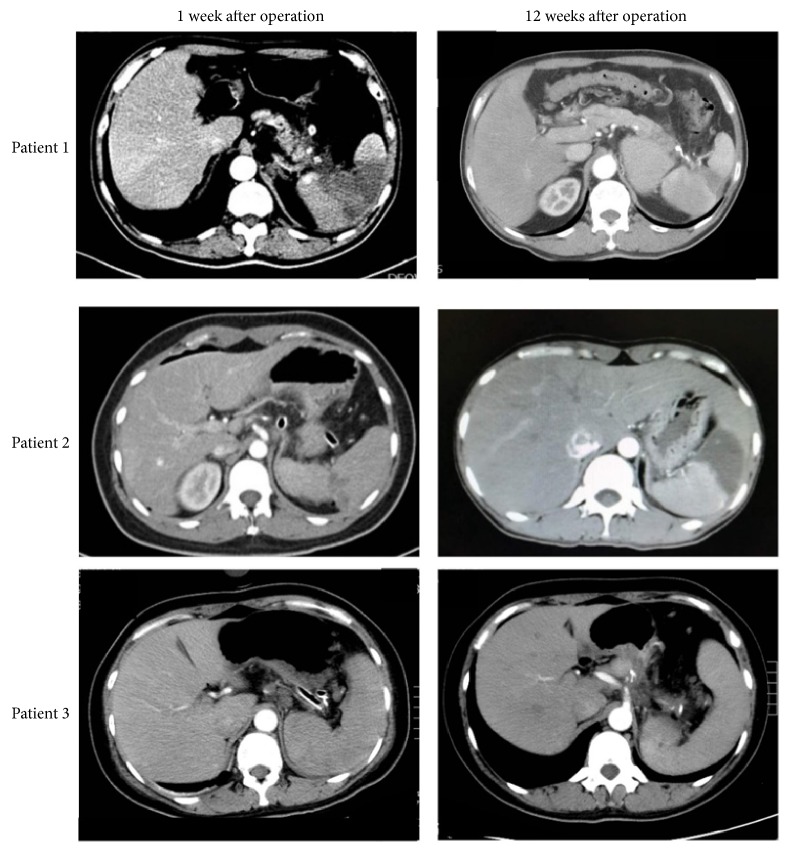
CT scanning images showing splenic infarction recovery during following up. Patient 1 had severe splenic infarction at 1 week after surgery. The infarction was obviously improved at week 12. The splenic infarction in patient 2 developed between the two follow-up visits. Patient 3 had only mild splenic infarction at week 1 and recovered completely after 12 weeks.
